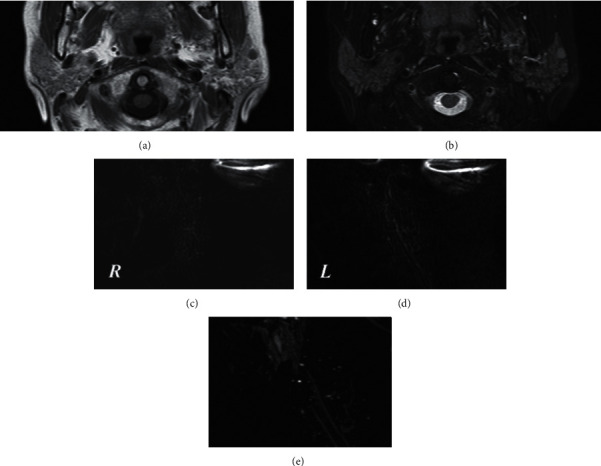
A case of the usefulness in determining the imaging side for unilateral sagittal 3D MR-S with T1- and T2-weighted images. T1-weighted image (a) and fat-suppressed T2-weighted image (b) showed HD of the parotid gland with left predominance. This case had no MHS in bilateral 2D MR-S (c, d). We determined the imaging side (right or left) for unilateral sagittal 3D MR-S by evaluating the HD on T1- and T2-weighted images (b, c). Unilateral left 3D MR-S (e) clearly depicted MHS. MR-S: magnetic resonance sialography; HD: heterogeneous distribution; MHS: multiple high-signal-intensity spots.
